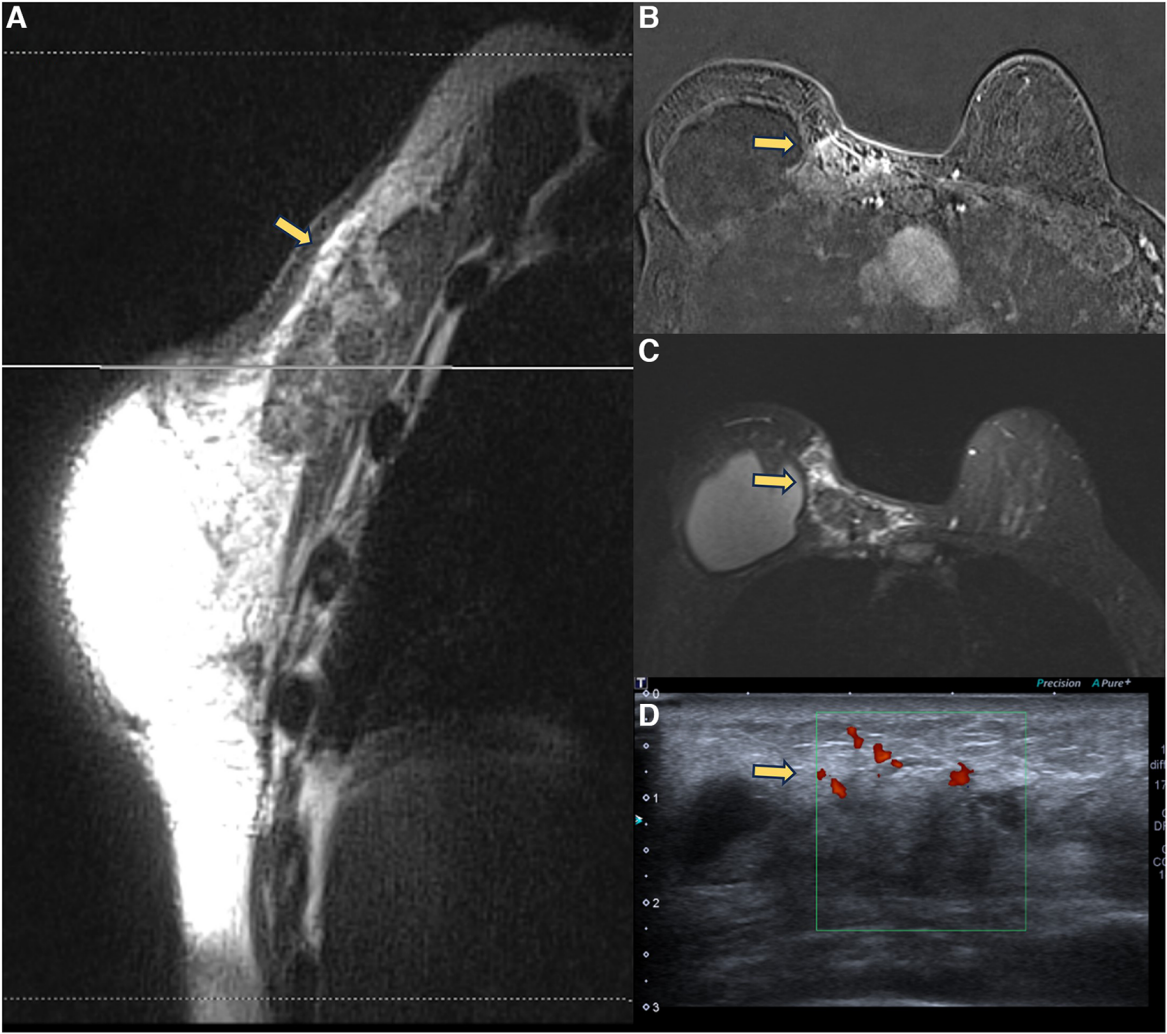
A 71-year-old woman with breast reconstructive surgery with implants for 5 years, presenting with a palpable mass in the medial quadrant. Sagittal proton density image (A), axial post-contrast imaging (B), axial T2 sequence (C), and ultrasonography show a heterogeneous mass with fat content and moderate enhancement, associated with skin thickening and without cleavage plan with the pectoral muscle. The ultrasound (D) shows a vascularized heterogeneous mass. The biopsy diagnosis was steatonecrosis.
